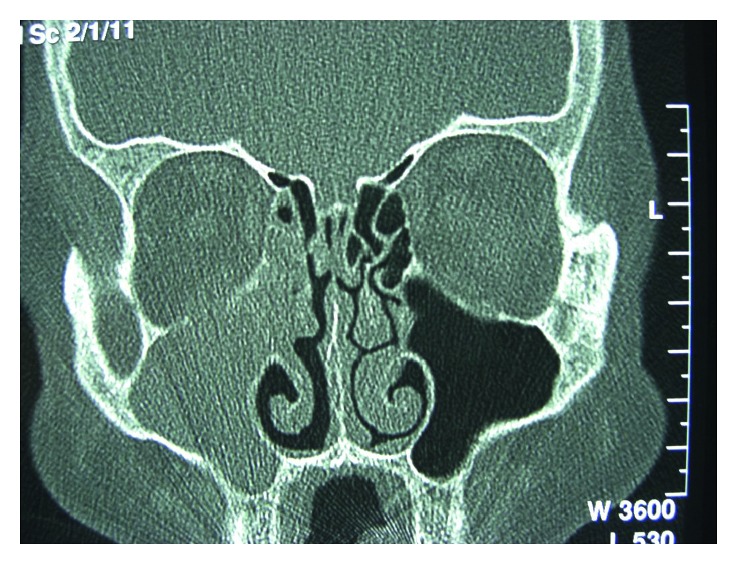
CT scan revealing an expansive mass occupying the right maxillary sinus (coronal view).
